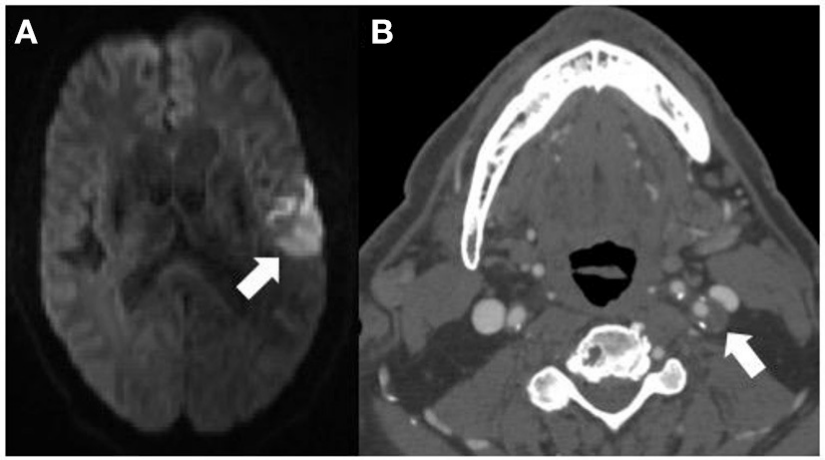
This 71-years-old patient presenting with an acute left middle cerebral artery territory infarction [arrow (A)] did not have any significant stenosis by North American Symptomatic Carotid Endarterectomy Trial criteria on CT angiography (CTA) and was thought to have an embolic stroke of undetermined source. The CTA (B) does however show a large, predominantly non–calcified plaque up to 5 mm in thickness in the proximal left internal carotid artery [(B) arrow] compatible with a vulnerable plaque, potentially the embolic source of the infarction.
